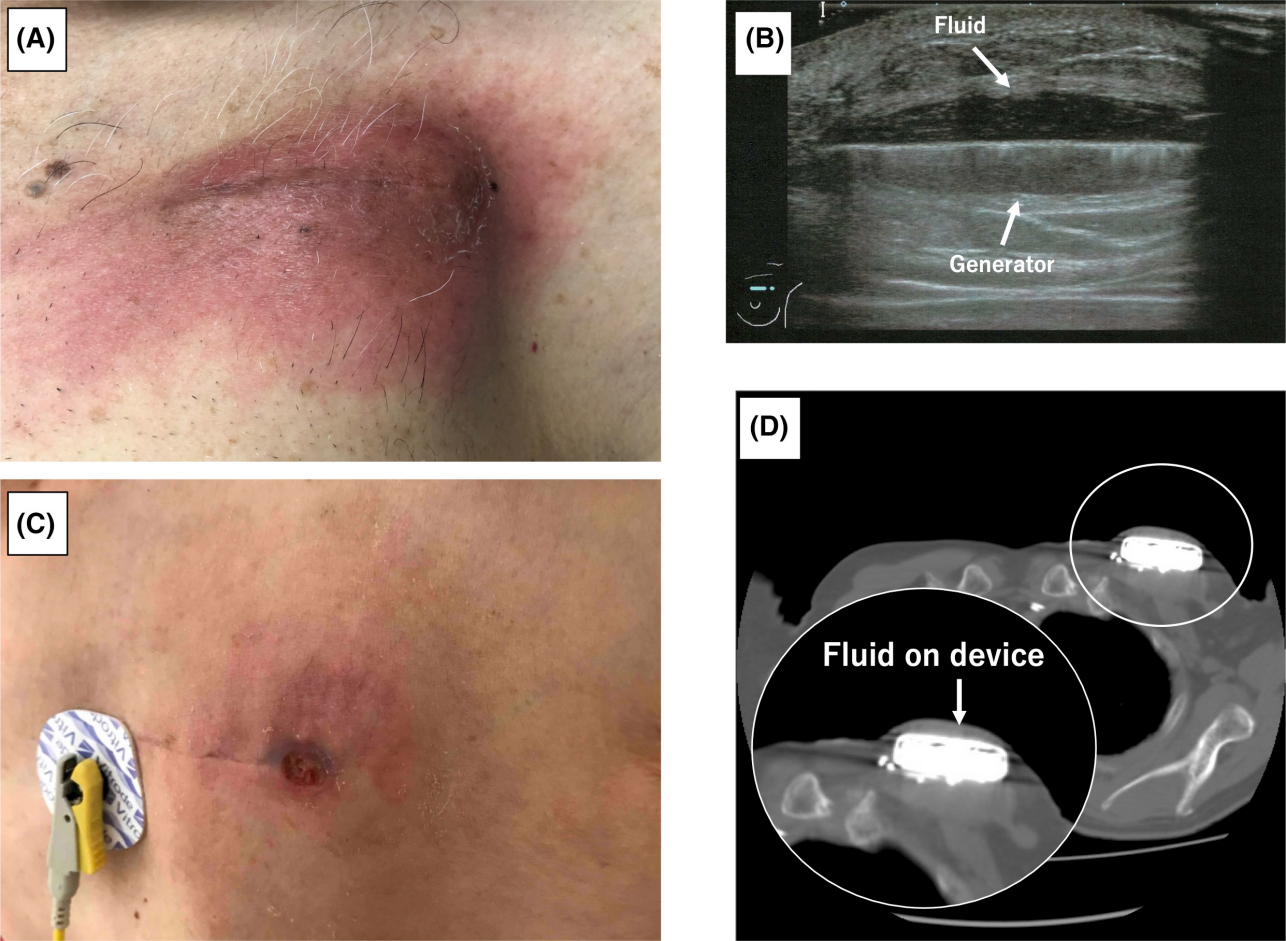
Images of the skin and sonographic or computed tomographic findings around the device‐implanted sites in Case 1 (A and B) and Case 2 (C and D).
